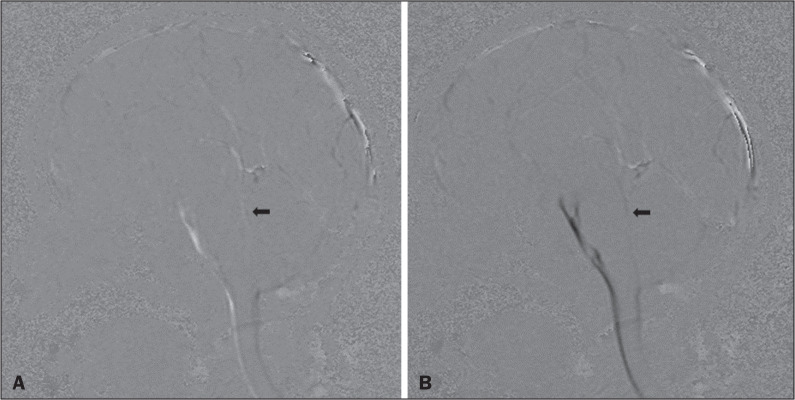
Qualitative sagittal cine-PC MRI sequences acquired during systole (A) and diastole (B) at the level of the cerebral aqueduct (arrows).
